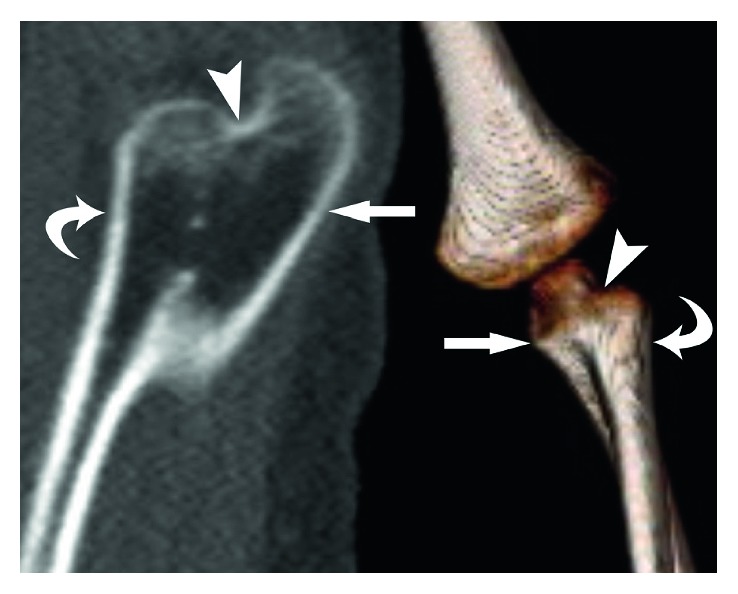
CT, sagittal reformatted (*left*) and 3D reconstructed (*right*) images demonstrate left radioulnar (radius = curved arrows, ulna = arrows) synostosis (arrowheads) in a patient with Klippel–Feil syndrome. (*Courtesy of Miguel Flores*, *MD*, *Orlando*, *FL.*)
